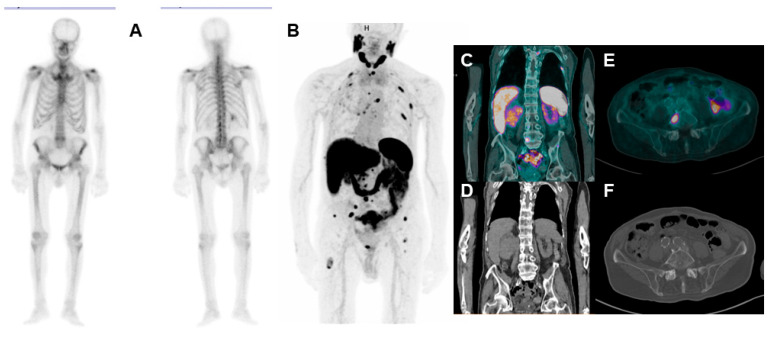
86-year-old CRPC patient undergoing bone scan ((A): anterior and posterior planar bone scan images) for rising PSA values (3.22 ng/mL). The scan shows no pathological uptakes; therefore, the patient underwent [^68^Ga]Ga-PSMA-11 PET/CT ((B): maximum intensity projection (MIP); (C,D): coronal-fused and CT images. (E,F): Axial-fused and CT images), with evidence of several bone focal uptakes, suggestive for metastases. Moreover, the scan shows a large area of increased uptake in the lower pole of the right kidney (SUVmax 6.8), associated with loco-regional lymph nodes with pathological uptake, compatible with renal SPN. In this case, a biopsy of bone lesions will be needed to discriminate their nature (PCa vs. RCC metastases).
